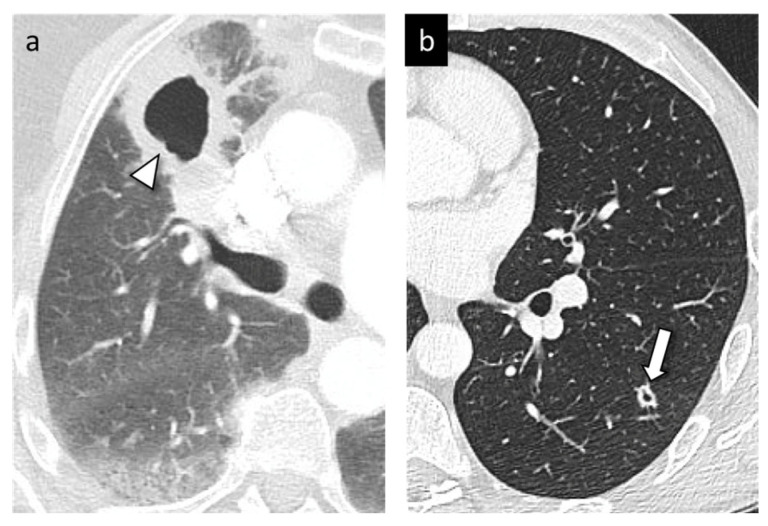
CT axial images of pulmonary nodules with cavitation. (a) 60-year-old woman with right upper lobe cavitary mass (arrowhead), consistent with PLSCC. (b) 48-year-old man with squamous cell carcinoma of the tongue and left lower lobe cavitary nodule, consistent with metastasis (arrow).
